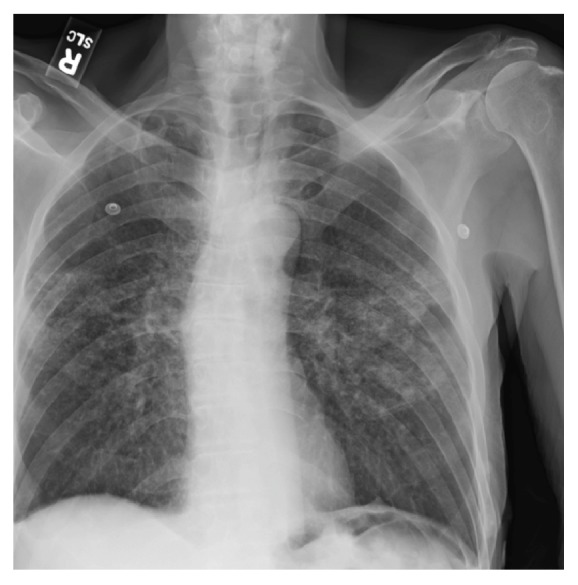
Posteroanterior chest X-ray demonstrating extensive ill-defined bilateral parenchymal opacities in the perihilar, mid, and lower lung zones.
